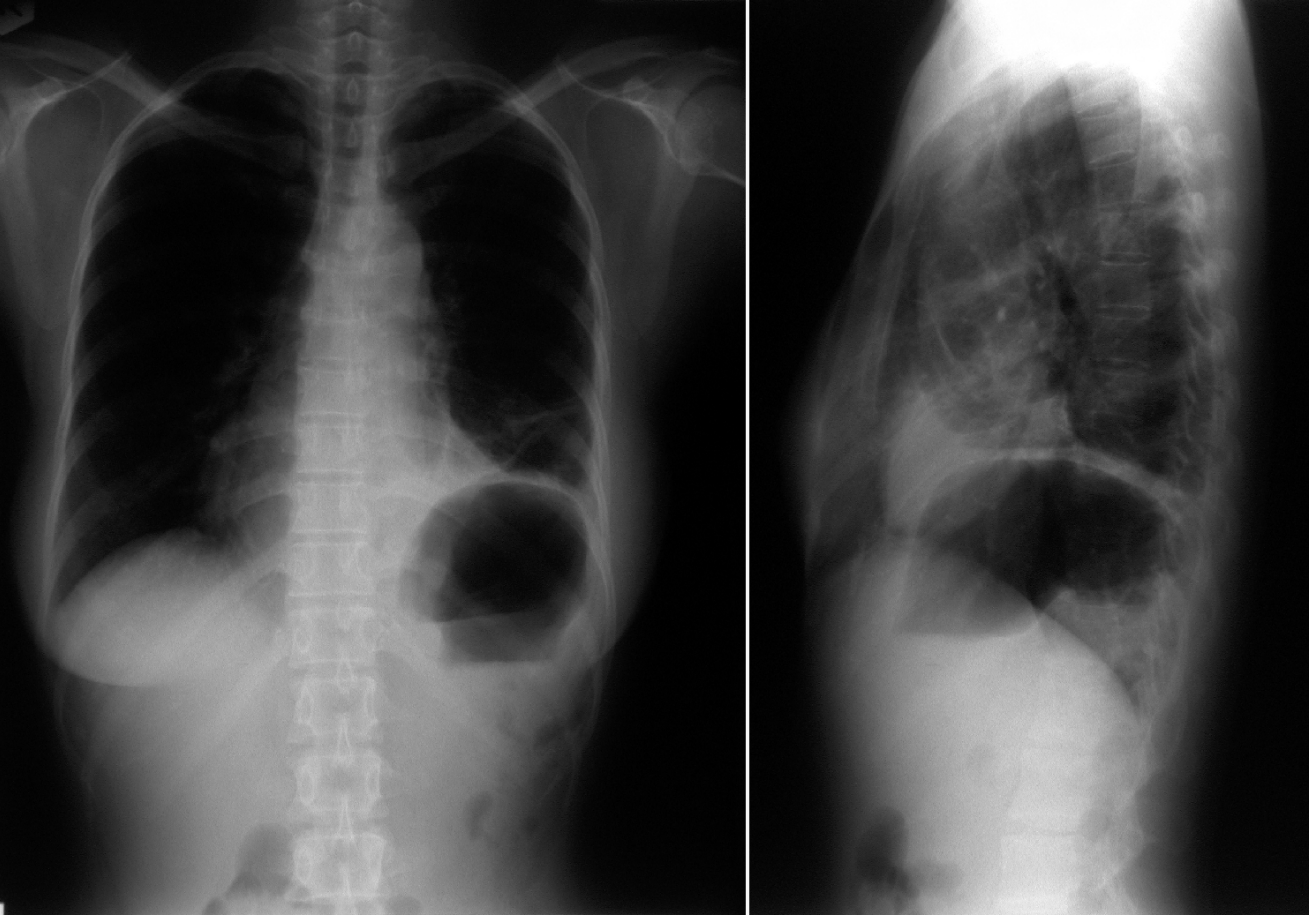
Postoperative X-ray of the chest after total resection of the teratoma, showing re-expansion of the left lung.
